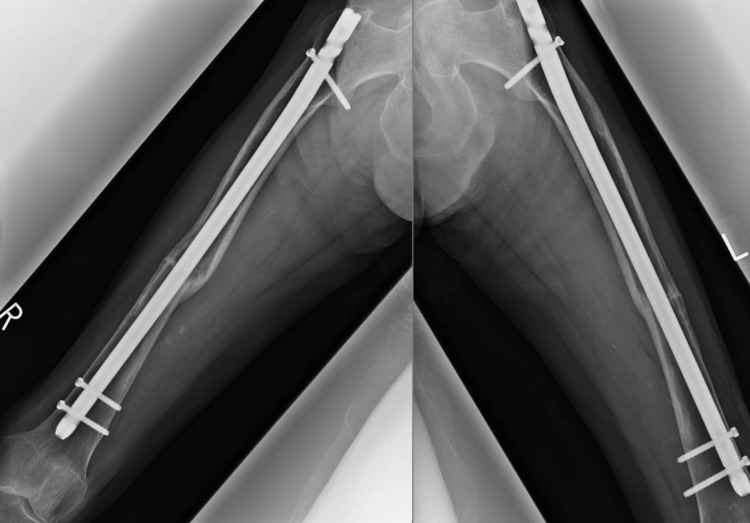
Plain radiographs of the right and left femurs one year after surgery when bone union was observed.
